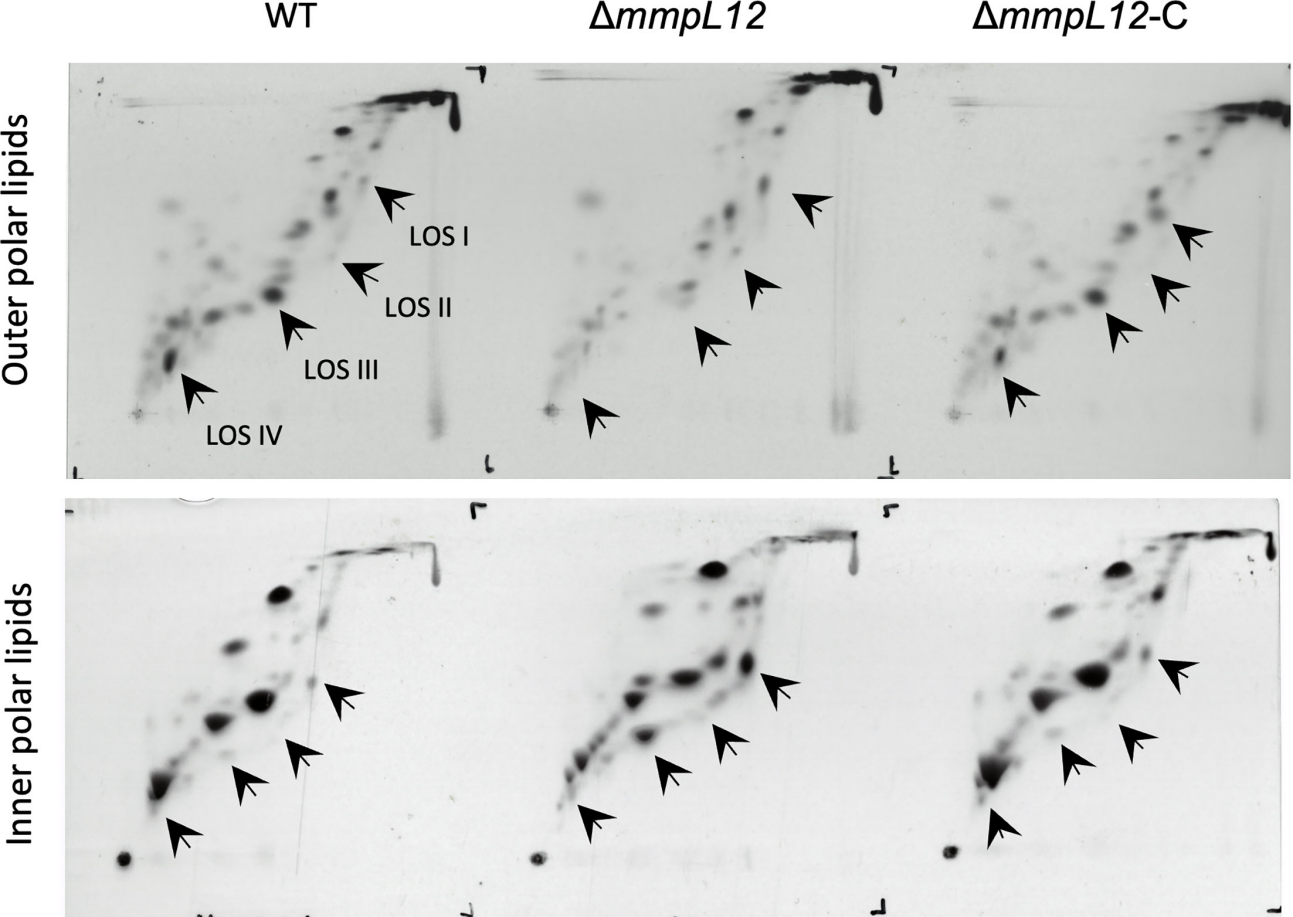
2D-TLC analysis of ^14^[C]-labelled polar lipids from *M. marinum* strains. Outer polar and apolar lipids were extracted first using water-saturated butanol from WT, mutant (Δ*mmpL12*) and complemented (Δ*mmpL12*-C) strains, followed by the extraction of inner polar lipids. Lipid species were separated using solvent system E (direction 1, CHCl3:CH3OH:H2O [60:30:6]; direction 2, CHCl3:CH3COOH:CH3OH:H2O [40:25:3 6]) and visualized on X-ray films by autoradiography. LOS species are indicated by arrows (for the mutant strain, arrows pointing to empty spots indicate putative positions where a LOS species would be expected to migrate). Set of TLCs represents one of two repeats.
